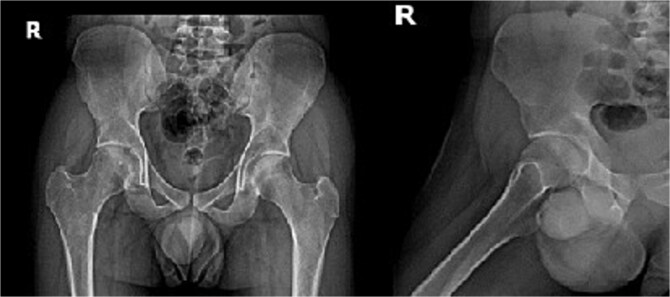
Imaging findings: Radiographs AP and lateral: Inconclusive findings of hip pathology.
